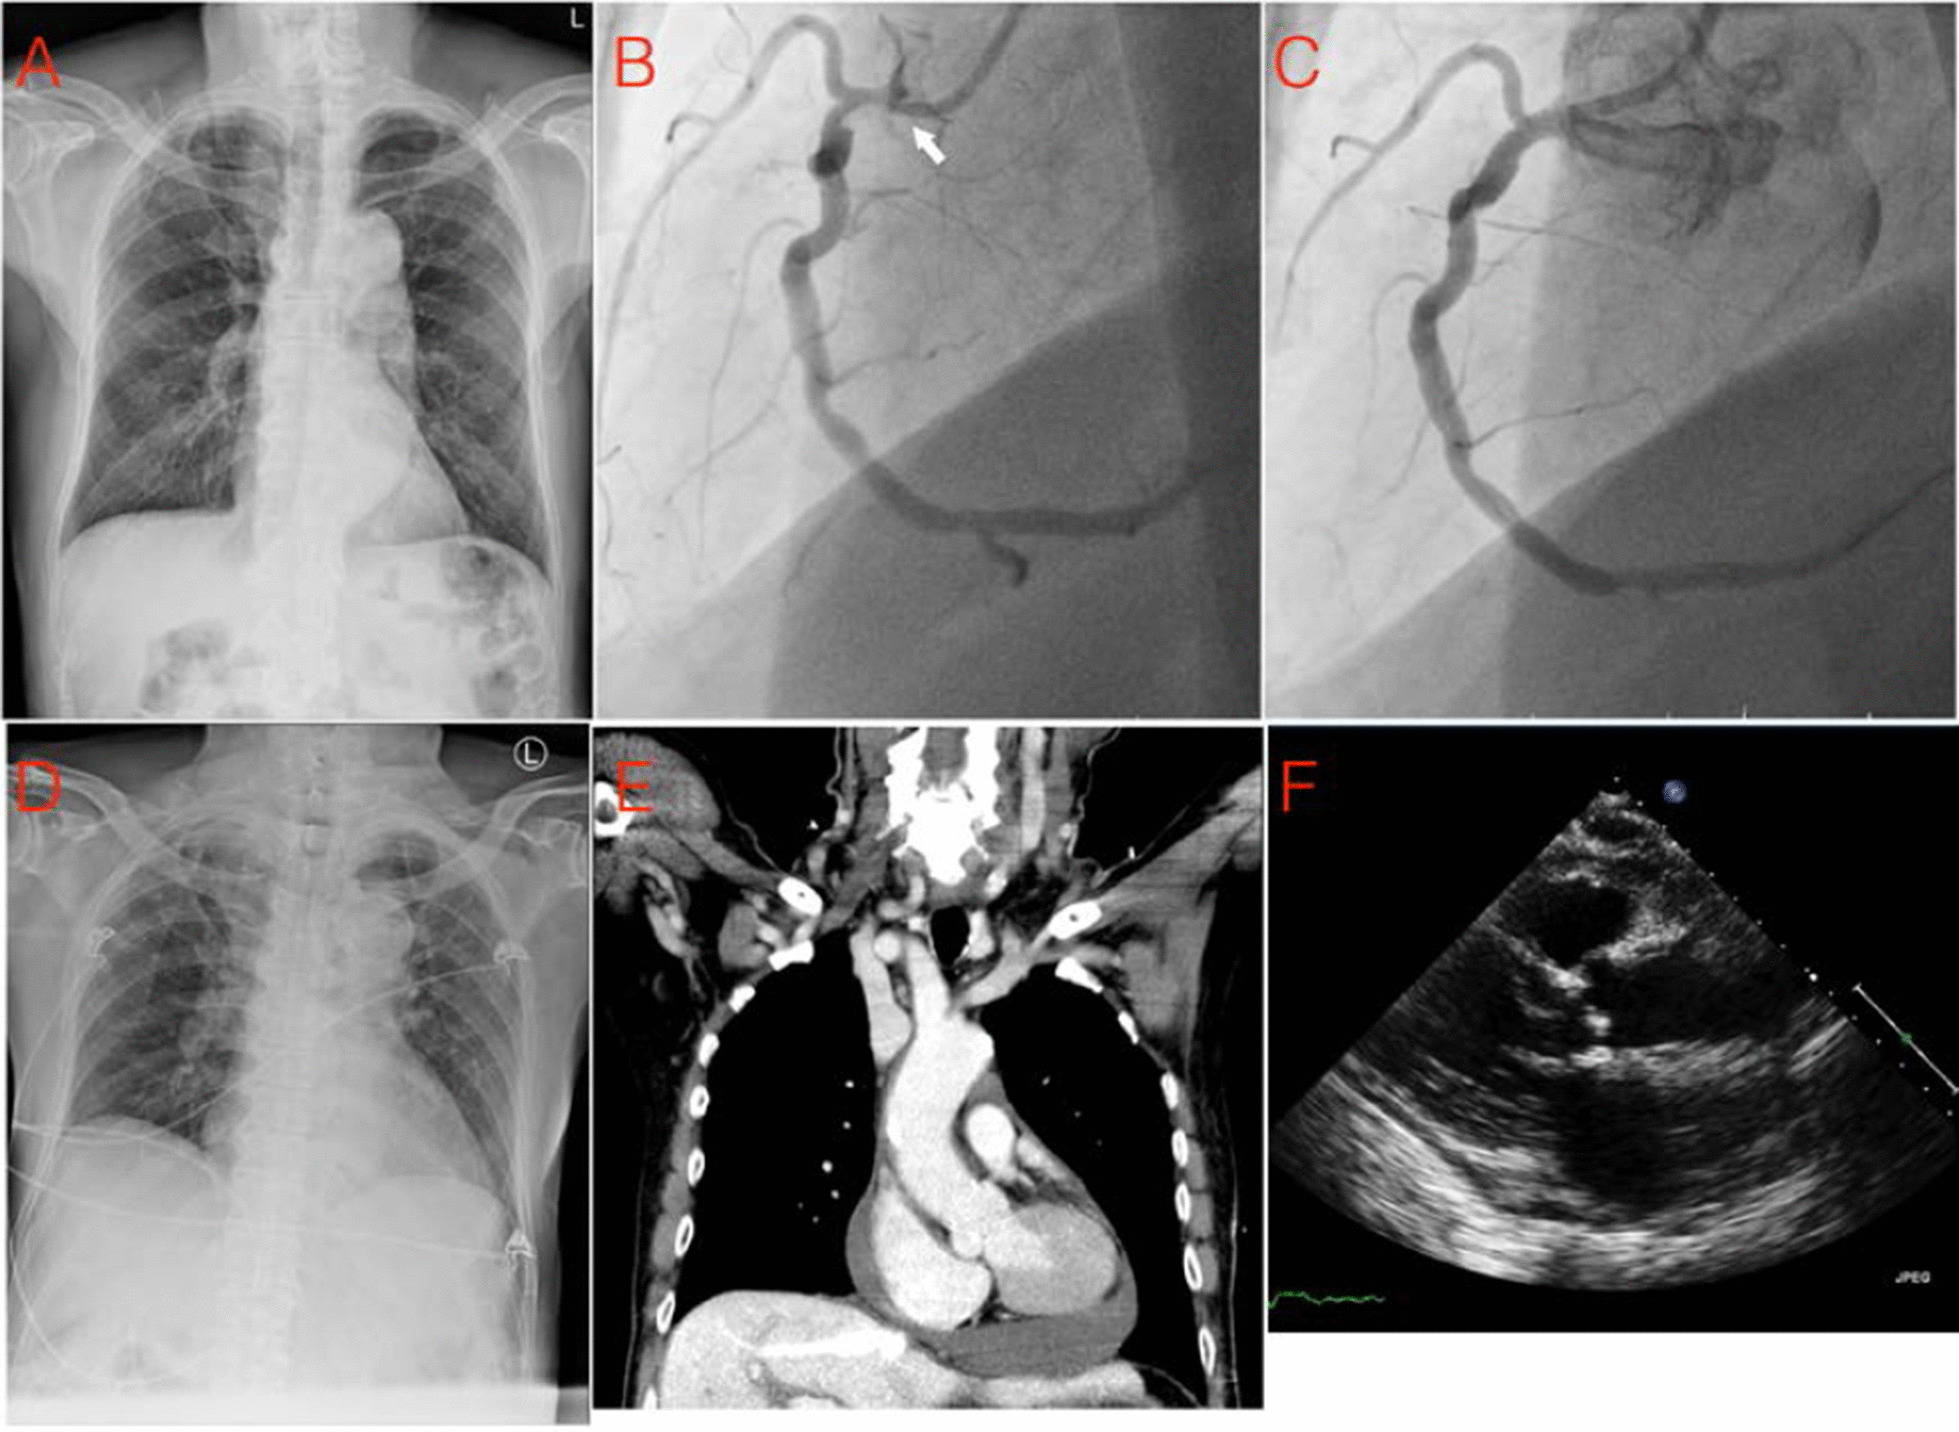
Case 2 series images. a Normal chest radiography (CXR) before intervention; b White arrow: narrow portion of right coronary artery orifice; c Stent deployed at orifice, no extravasation found; d CXR at 2 h after stent deployment; e chest computed tomography (CT) showed no dissection, but a significant amount of pericardial effusion; f Follow-up echocardiography after steroid treatment showed decreased pericardial effusion without signs of RV collapse
